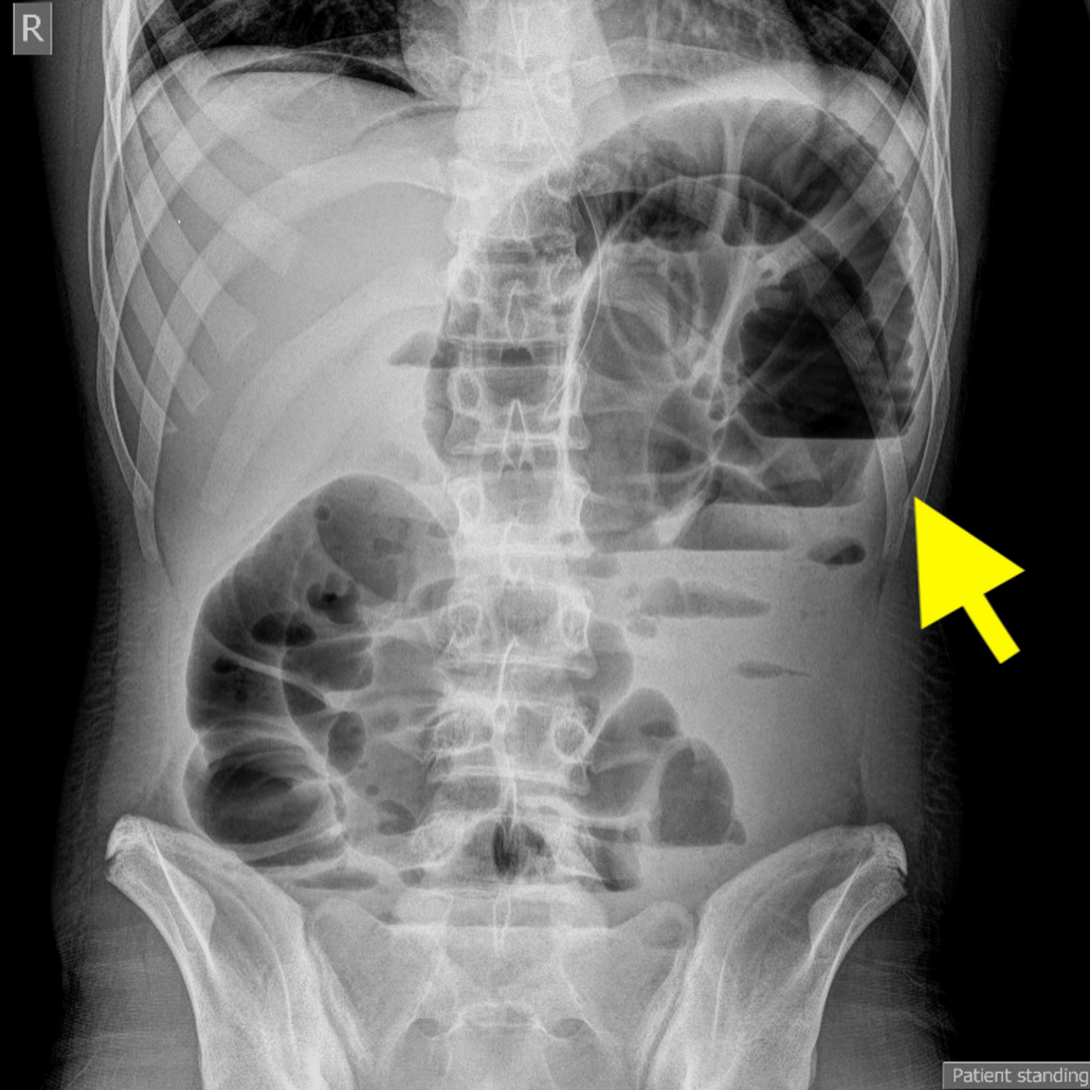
Presence of ileus in abdominal X-ray image. The arrow points to the abdominal distension (POD 5). POD: postoperative day
